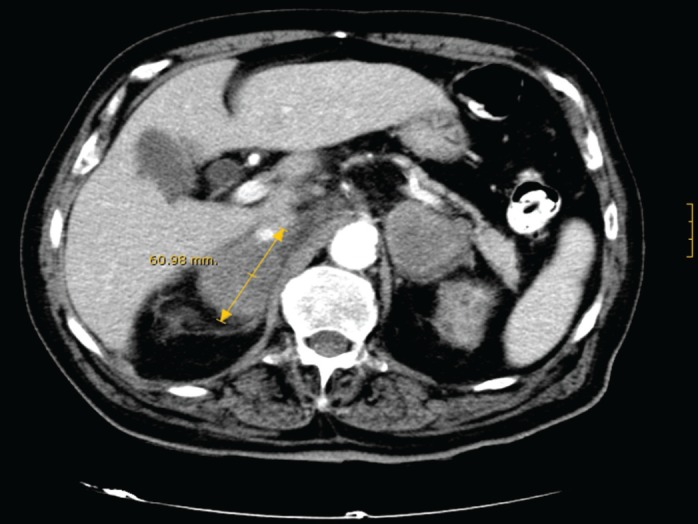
CT-scan showing a bilateral heterogeneous mass of about 6 cm in diameter in both adrenal glands.
